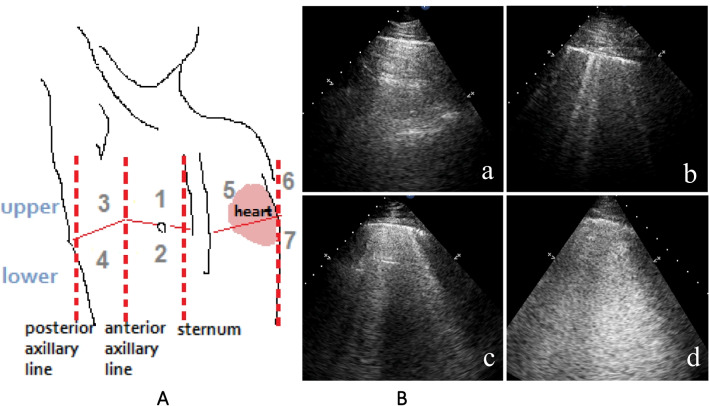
Ultrasound lung comets (ULCs) scoring method. A Seven-zones: the anterior zone was delineated from the sternum to the anterior axillary line, and the lateral zone was from the anterior to the posterior axillary line. The anterior and lateral chest walls were subdivided into upper and lower halves with the fourth intercostal space as the boundary from the clavicle to the diaphragm anterior (lower area on the left side was subsequently removed because most of the study population had an enlarged heart which intervened with the area). B Increasing severity of interstitial or alveoli involvement. (a) Normal lung = 0: ULCs are absent. (b) Septal syndrome = 1: ULCs are about 7 mm apart, corresponding to subpleural septa. (c) Interstitial-alveolar syndrome = 2: ULCs are confluent. (d) White lung = 3: ULCs have coalesced, resulting in an almost completely white echographic lung field [14]
